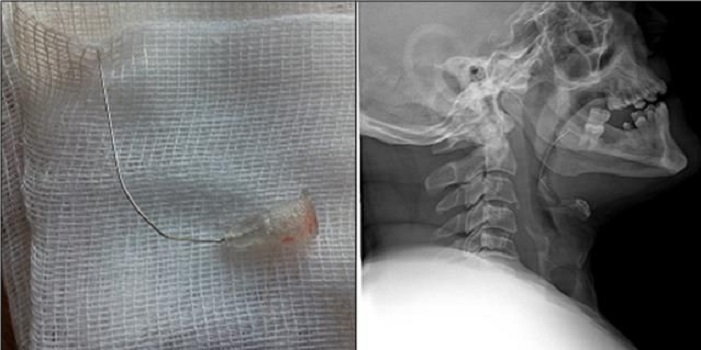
X-Ray and foreign body's of Case 1
